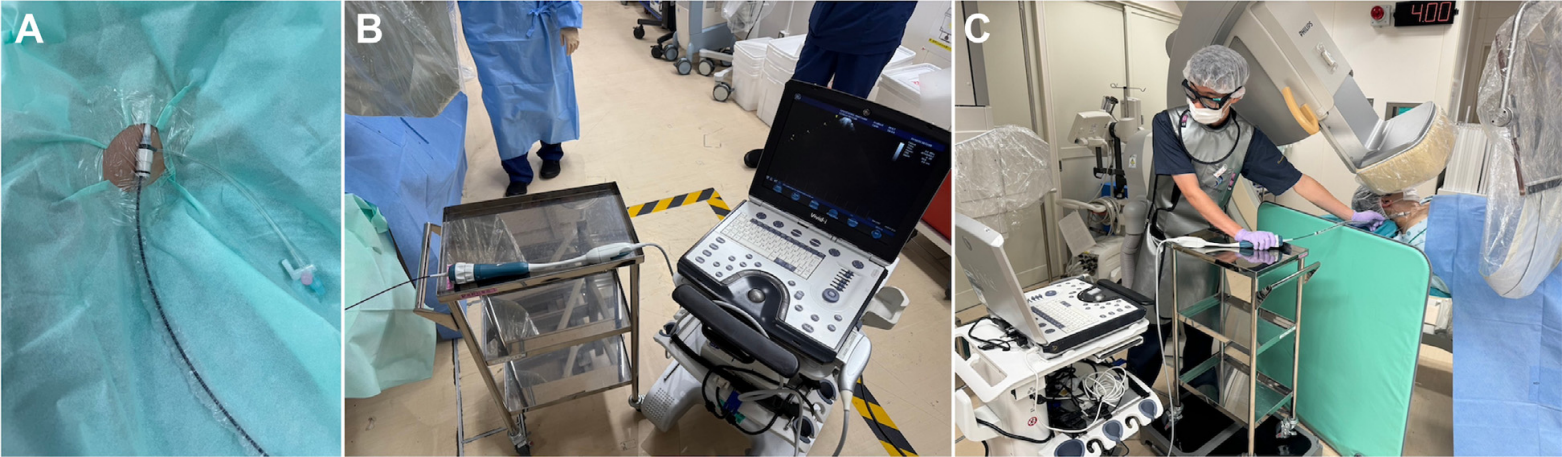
Puncture Site and Intracardiac Echocardiography Setup (A) Intracardiac echocardiography catheter with a sterile sleeve. The use of a sterile sleeve and a transparent seal maintains cleanliness of the puncture site and internal tissues while allowing the echocardiographer to operate in a nonsterile environment. (B) Intracardiac echocardiography device on a table. Placing the intracardiac echocardiography device on a table at the same height as the puncture site enhances stability during the procedure. (C) Echocardiographer operating an intracardiac echocardiography device. Reducing radiation exposure for the echocardiologist is crucial, in addition to maintaining sterile technique. Our institution uses a radiation shield of appropriate size to avoid interference with fluoroscopic equipment.b.Manipulate ICE to obtain views:i.Long-axis ascending aorta view (Figure 7A):•Advance the catheter about 5 cm from the tip of the sheath with clockwise or counterclockwise rotation.•Evaluate the ascending aorta before the procedure.
